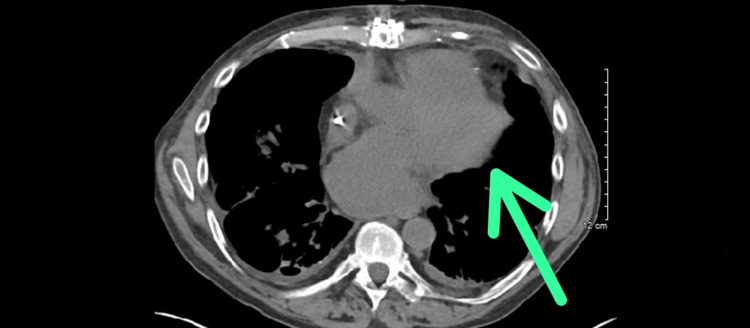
Cross-sectional view of the repeat CT of the chest without contrast showing improvement in the previously seen left pericardial fat opacity (green arrow) CT: computed tomography
